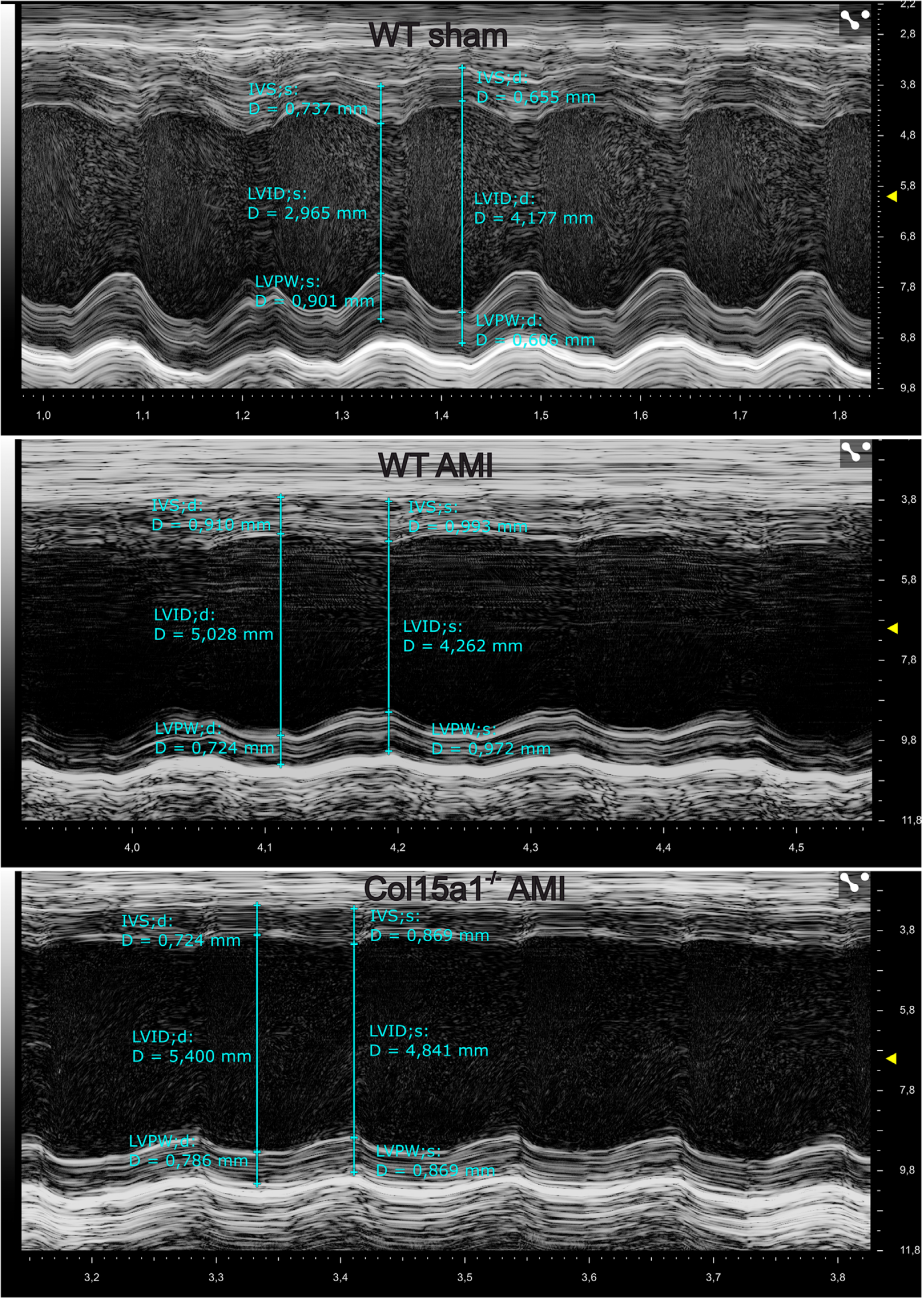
Representative transthoracic M‐mode SAX images showing the measurement of echocardiography parameters. Images of wild‐type sham (noninfarcted) (uppermost figure), wild‐type AMI (middle figure) and *Col15a1*
